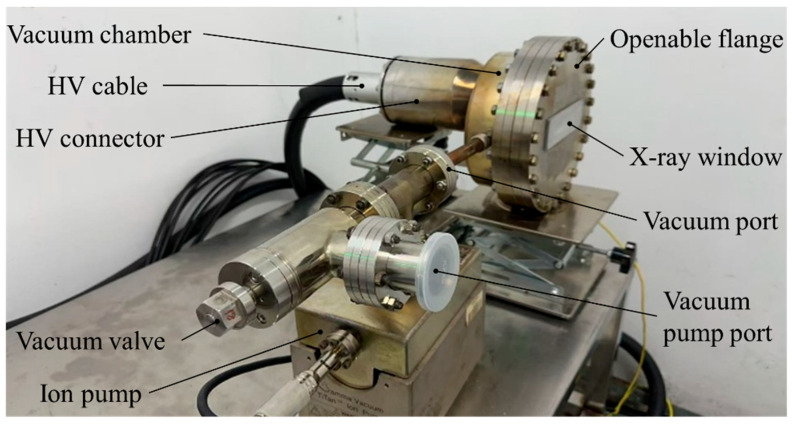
X-ray experimental tube.
